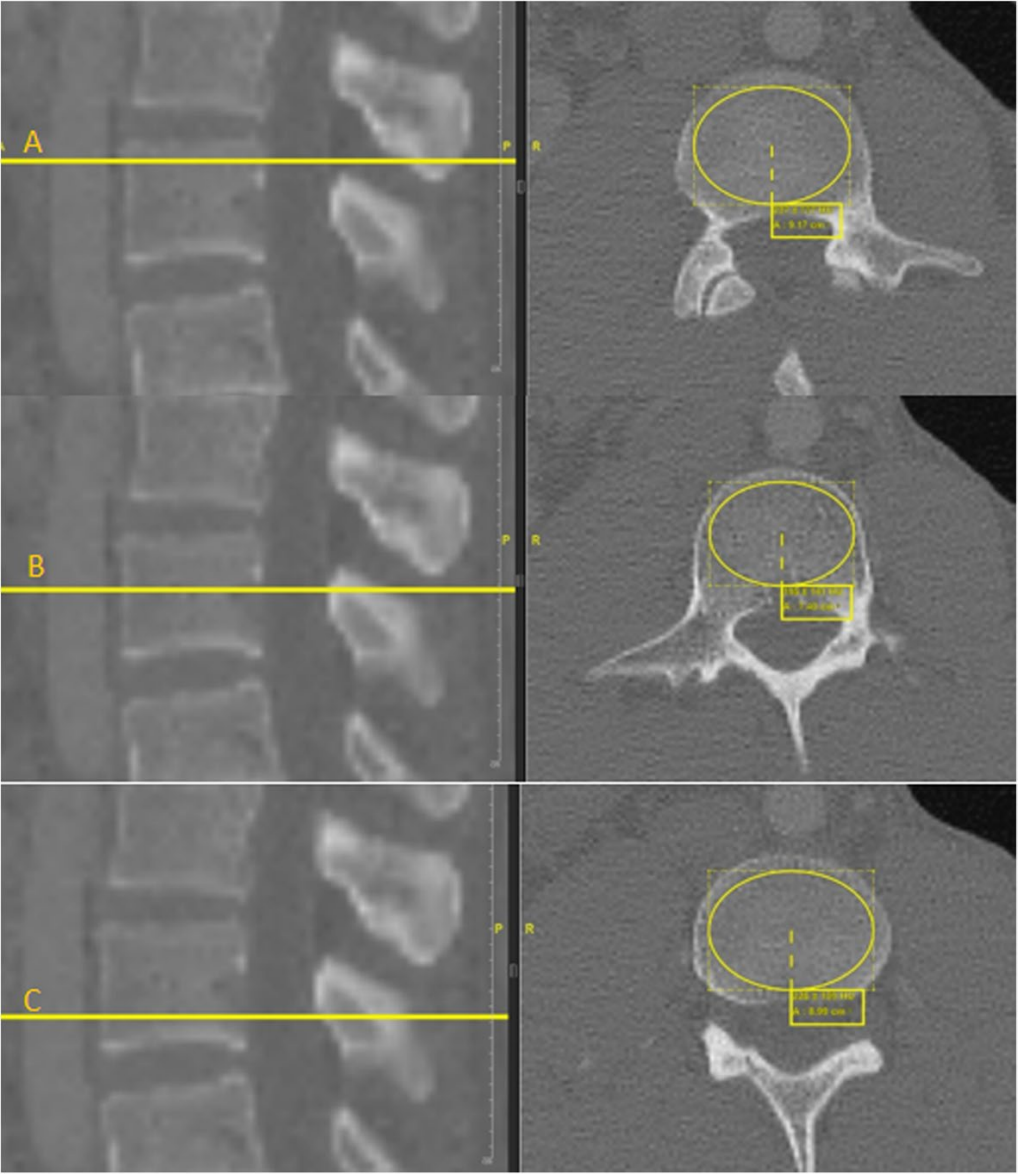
Determination of the HU of the L3 vertebral body in 3 localizations below the upper plate A, in the middle of the vertebral body B and above the base plate C using contrast-enhanced computed tomography
